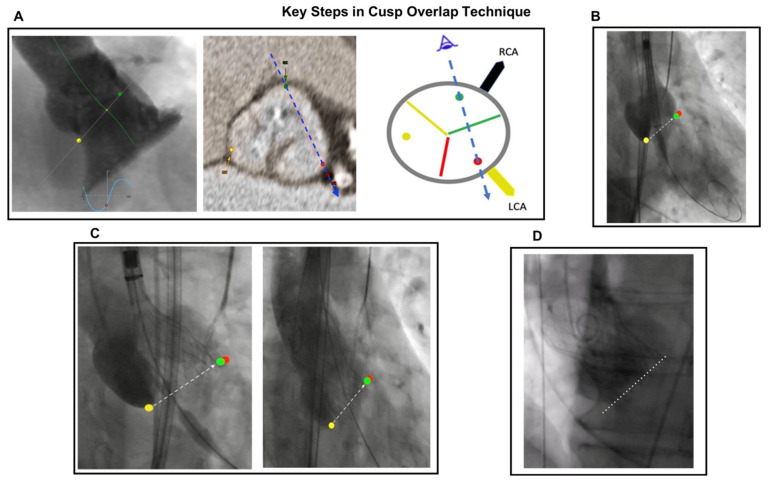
Key Steps in Cusp-Overlap Technique. (A) Step 1: a reconstructed computed tomography angiography overlay of the cusp-overlap view. (B) Step 2: a fluoroscopic view in RCC-LCC cusp overlap with Safari2 Wire appropriate in Left Ventricle and Implantation Starting Position. (C) Step 3: a fluoroscopic image demonstrates a 3 mm depth in the cusp-overlap view after full annular contact below the non-coronary cusp. (D) Step 4: a final aortography performed in the cusp-overlap view. Yellow color: Non Coronary Cusp (NCC); green color: Right Coronary Cusp (RCC); red color: Left Coronary Cusp (LCC); blue color: angle view, Dashed line: Annulus marker.
